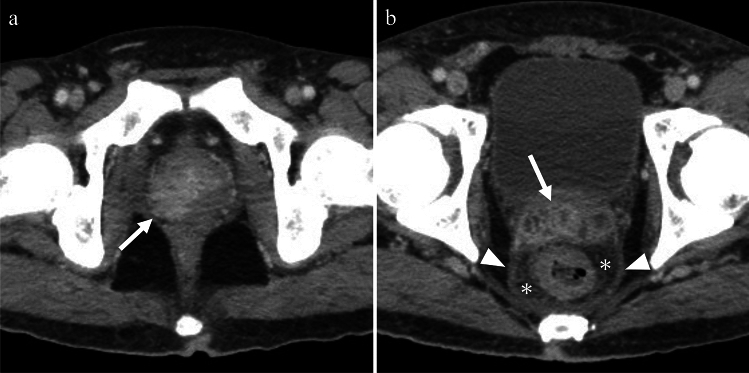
Man in his 30 s with leukemia presenting with fever with unknown causative pathogen. a The prostate is enlarged and shows marked enhancement predominantly on the right side (arrow) on contrast-enhanced CT, indicating prostatitis. b Contrast-enhanced CT showing diffuse wall and septal thickening of the seminal vesicle (arrow), thickening of the mesorectum (arrowhead), and increased haziness of the perirectal fat plane (asterisks). These indicate seminal vesiculitis
